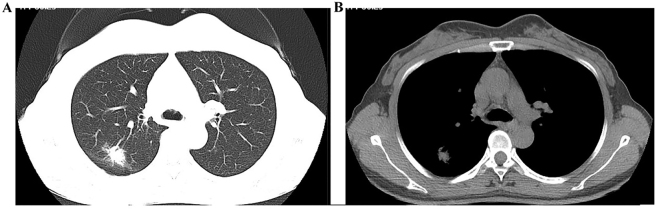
Chest CT scan demonstrating a right upper-lobe pulmonary nodule. (A) Lung window; (B) mediastinal window.
